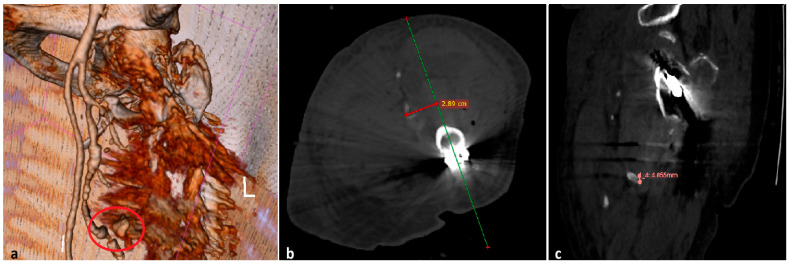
Angio-CT image investigation: (a) vascular injury associated with contrast medium extravasation (red circle); (b) the distance between the screw trajectory (green line) and the profunda femoris artery lesion, axial view (red line); (c) the distance between the vascular lesion and screw direction, sagittal view.
